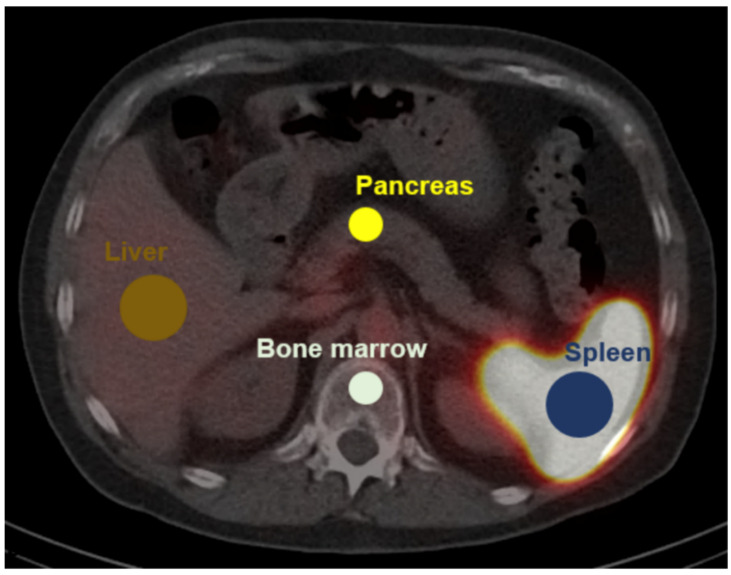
VOI definition for quantification of physiologic uptake. ^99m^Tc-labelled denatured red blood cells single photon emission tomography (SPECT) is fused on low dose computed tomography (CT). Diameter of spherical 3D-VOIs: pancreas 1.5 cm, bone marrow 1.5 cm, spleen 3.0 cm, liver 3.0 cm.
